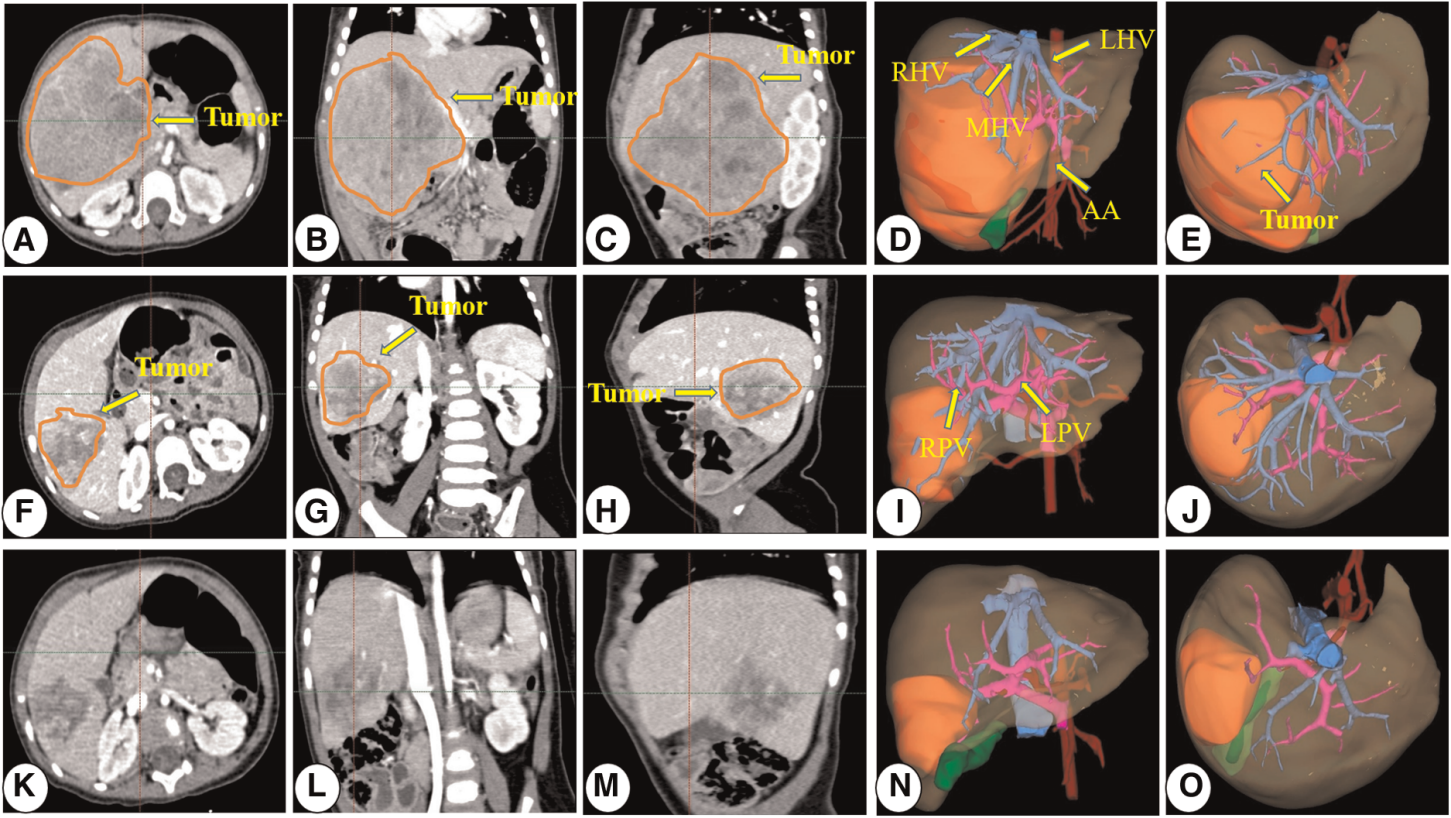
Representative preoperative chemotherapy assessment of a patient who underwent neoadjuvant chemotherapy before surgical resection. (A–E) CT images and 3D reconstruction images at diagnosis; (E–J) CT images and 3D reconstruction images after two courses of preoperative chemotherapy; (K–O) CT images and 3D reconstruction images after three courses of preoperative chemotherapy. CT, computed tomography; 3D, three-dimensional; RPV, right portal vein; LPV, left portal vein; PV, portal vein; RHV, right hepatic vein; LHV, left hepatic vein; MHV, middle hepatic vein; AA, abdominal aorta.
